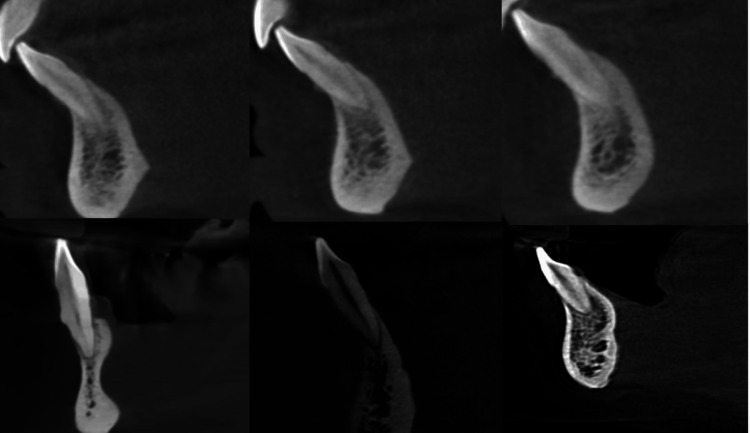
Multiple CBCTs depicting single canal or type 1 Vertucci configuration. CBCT - Cone beam computed tomography
